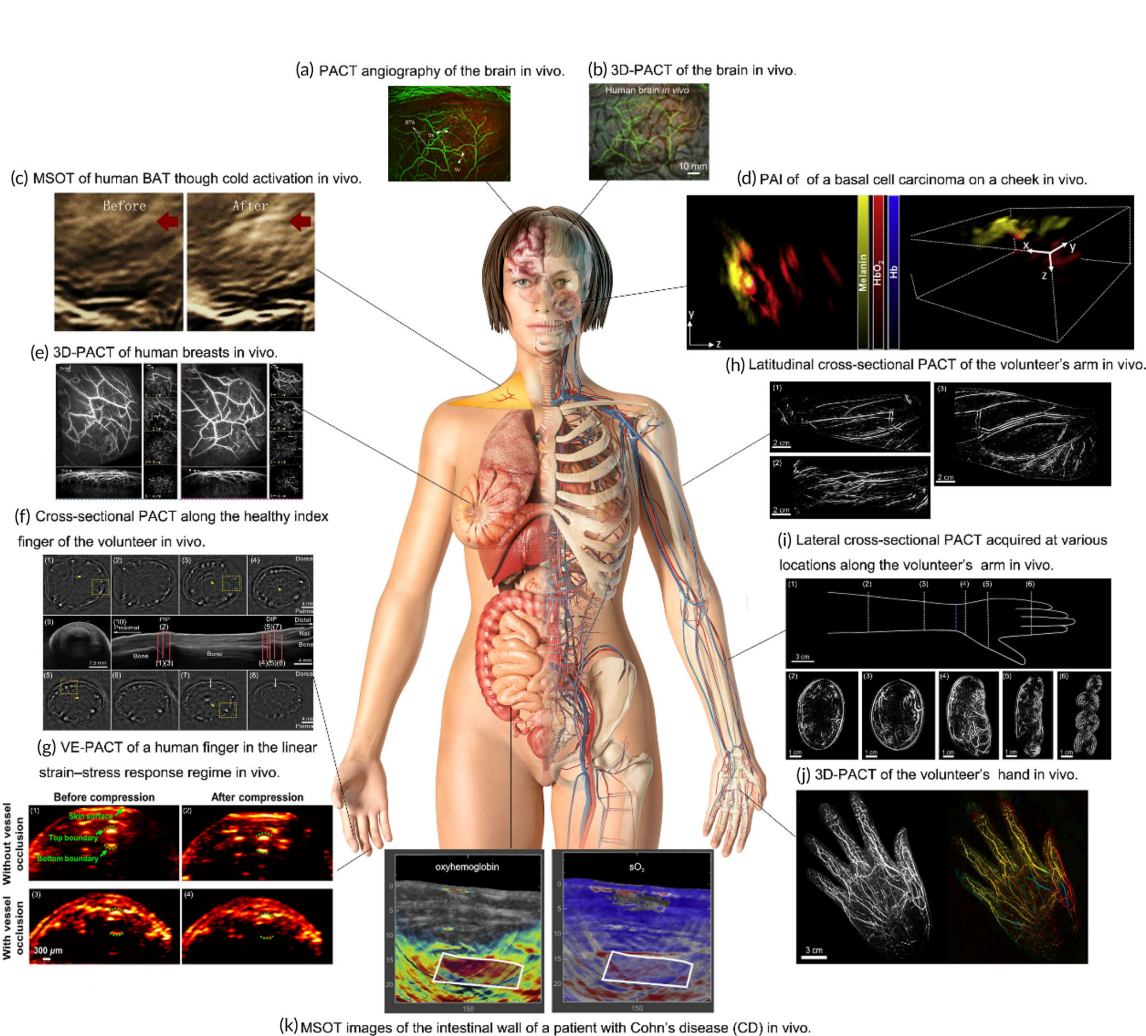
Photoacoustic computed tomography (PACT) imaging of various organs and parts of the human body. (a) PACT angiography of the brain in vivo.^34^ Images are segmented into scalp (green) and cortical (red) regions. (b) 3D‐PACT image of the brain in vivo.^109^ (c) Multispectral optoacoustic tomography (MSOT) images of human brown adipose tissue (BAT) before and after cold exposure.^104^ (d) PACT image of a basal cell carcinoma on the cheek skin with cross‐sectional view on the left and 3D rendering of the lesion on the right.^120^ (e) 3D‐PACT images of human breasts in vivo.^100^ The upper left figure is a fluoroscopic angiogram of the right breast in a healthy person, the lower left figure is a maximum amplitude projection (MAP) image of the right breast in lateral view, and the four smaller images on the left are cross‐sectional images of the right breast from the nipple to the chest wall in different coronal planes. Each cross‐sectional image is a 1 cm thick MAP of the breast section. The right figures are the left breast of the same person. (f) Cross‐sectional PACT images along the healthy index finger of the volunteer in vivo, which show the blood vessels along the length of the finger as the intense white areas.^25^ Subfigures (1)–(8) show eight cross sections, containing images across the proximal interphalangeal point joint, distal interphalangeal point joint, and the nail wall, in approximately the same locations as those marked in the ultrasound images. (g) Vascular elastic PACT images of a human finger in linear strain–stress response regime in vivo.^110^ Subfigures (1) and (2) show comparative cross‐sectional PACT images of a human finger without vascular occlusion before and after compression, and subfigures (3) and (4) show corresponding PACT images with vascular occlusion before and after compression. (h) Latitudinal cross‐sectional PACT of a volunteer's arm in vivo.^29^ The three images are longitudinal cross sections of the upper right forearm, the lower right forearm (ending at the wrist), and the right biceps muscle of the volunteer. (i) Lateral cross‐sectional PACT images acquired at various locations along a volunteer's arm in vivo.^29^ (1) A sketch image of the arm. The white dotted line details the location of each cross‐sectional image in images (2)–(6). The blue dotted line marks the wrist, where the carpal bone meets the radius and ulna. (2) An image of the upper forearm, 10 cm above the wrist. (3) An image of the lower forearm, 3 cm above the wrist. (4) An image of the palm, 1 cm below the wrist. (5) An image of the middle of the palm, 3 cm below the wrist. (6) An image of the finger (excluding the thumb), approximately midway between the proximal phalanges. (j) 3D‐PACT images of the volunteer's hand in vivo.^29^ The MAP of the PA signal along the Z‐axis is presented on the left, and a color‐coded depth image of the hand is presented on the right. (k) MSOT images with increased signals for oxyhemoglobin and sO_2_ in the intestinal wall of a patient with Cohn's disease.^111^
